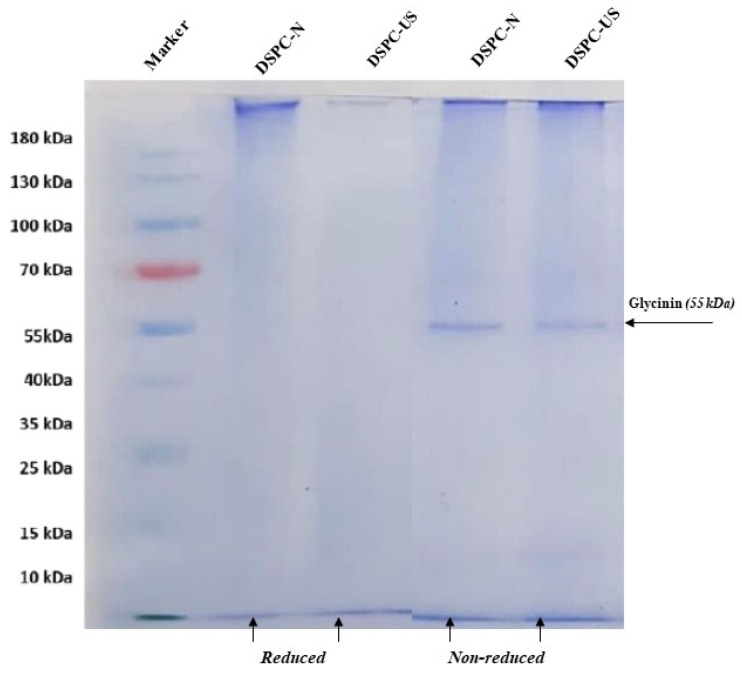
SDS-PAGE electrophoretic protein profiles of native (DSPC-N) and ultrasound applied (DSPC-US) date seed protein concentrate under reducing and non-reducing conditions.
